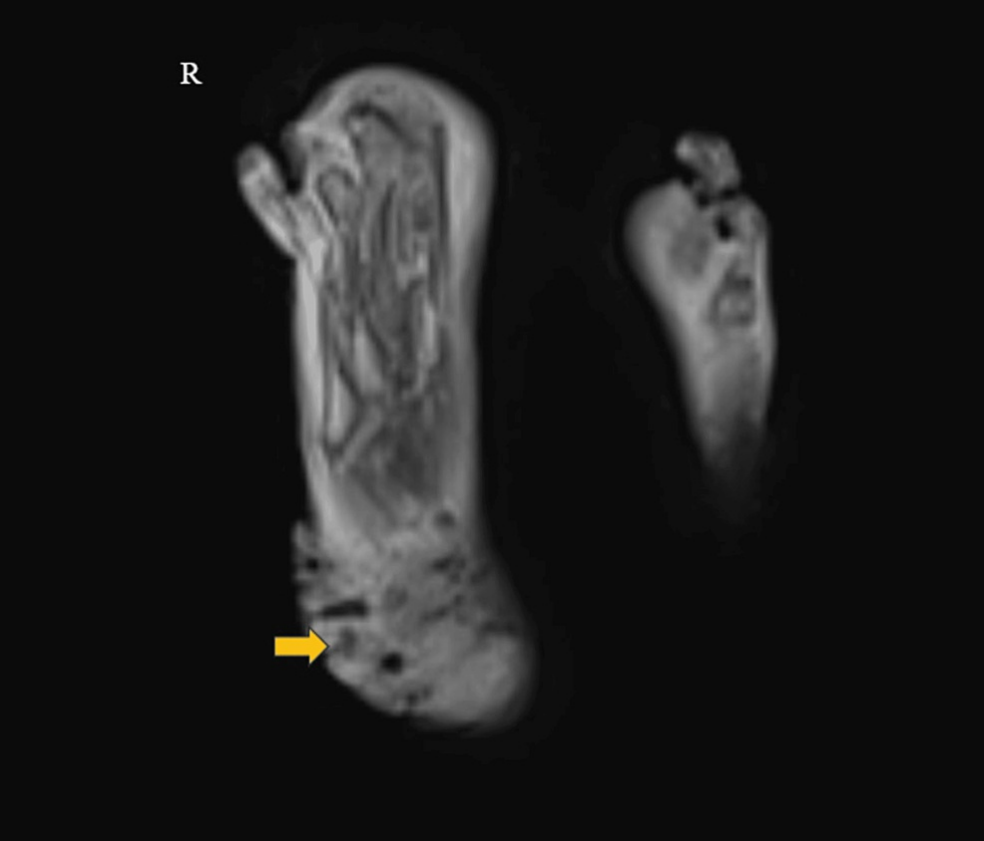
An MRI of the right lower limb axial T1WI shows cerebriform connective tissue overgrowth on the plantar and lateral aspects of the right foot (yellow arrow). T1WI: T1-weighted imaging
